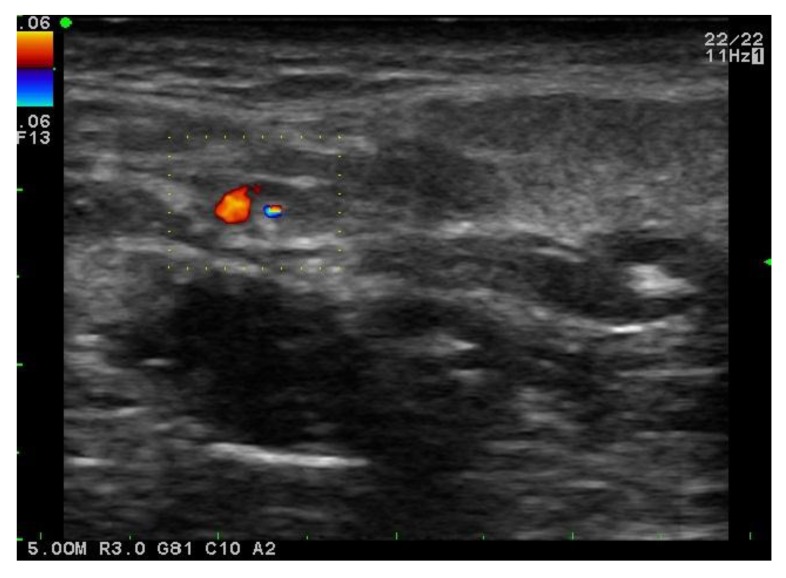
Doppler US: right upper cervical lymph node with hilar vascularity.
